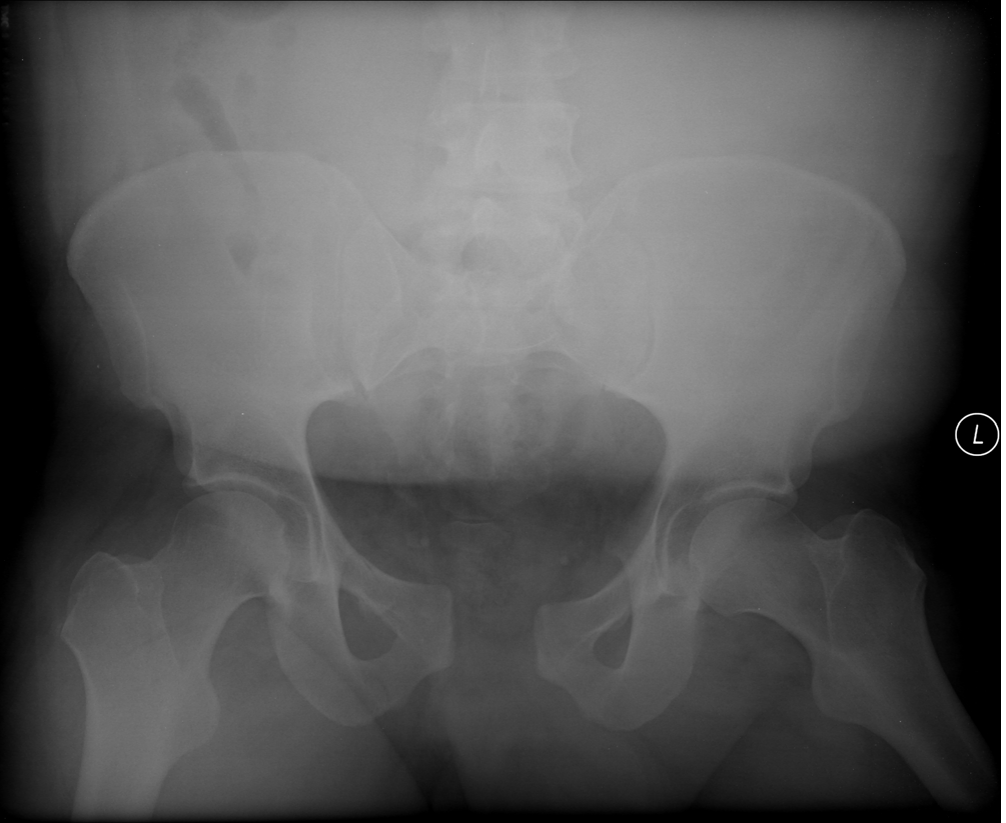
Presenting AP pelvis radiograph demonstrating the open-book pelvic fracture with right, nondisplaced superior and inferior rami fractures, symphyseal diastasis of 4 cm and complete disruption of the right sacroiliac joint.
